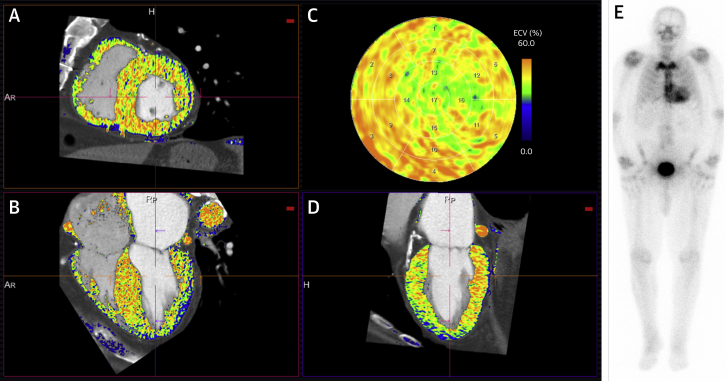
Automated ECV_CT_ Heart Model Output With Corresponding 3-h Planar DPD Image ECV_CT_ map superimposed on the CTCA images (A to D) and corresponding 3-h planar DPD image (E). The endocardial and epicardial contours can be edited in the short-axis (A), 4-chamber (B), and 2-chamber (D) views to produce an ECV_CT_ American Heart Association 17-segment polar map (C). This is a patient with aortic stenosis (AS) amyloid (Perugini grade 2 ^99m^Tc-3,3-diphosphono-1,2-propanodicarboxylic acid [DPD] scintigraphy) with total myocardial ECV_CT_ is globally elevated at 47%. Abbreviations as in Figure 1.
